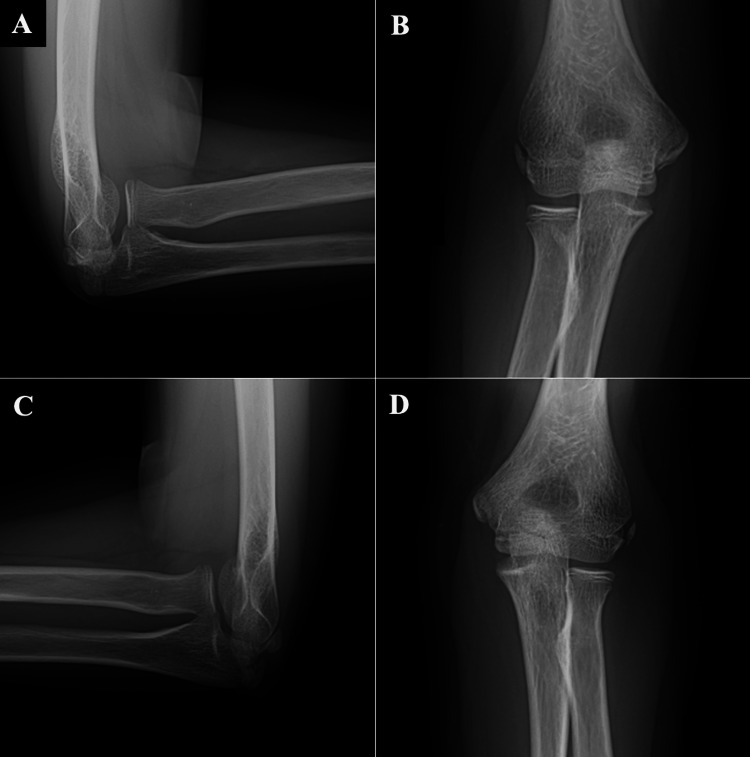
X-ray of right (A, B) and left (C, D) elbow at one year of follow-up. 4A shows a lateral radiographic view of the right elbow; 4B shows an anteroposterior radiographic view of the right elbow; 4C shows a lateral radiographic view of the left elbow; 4D shows an anteroposterior radiographic view of the left elbow.
